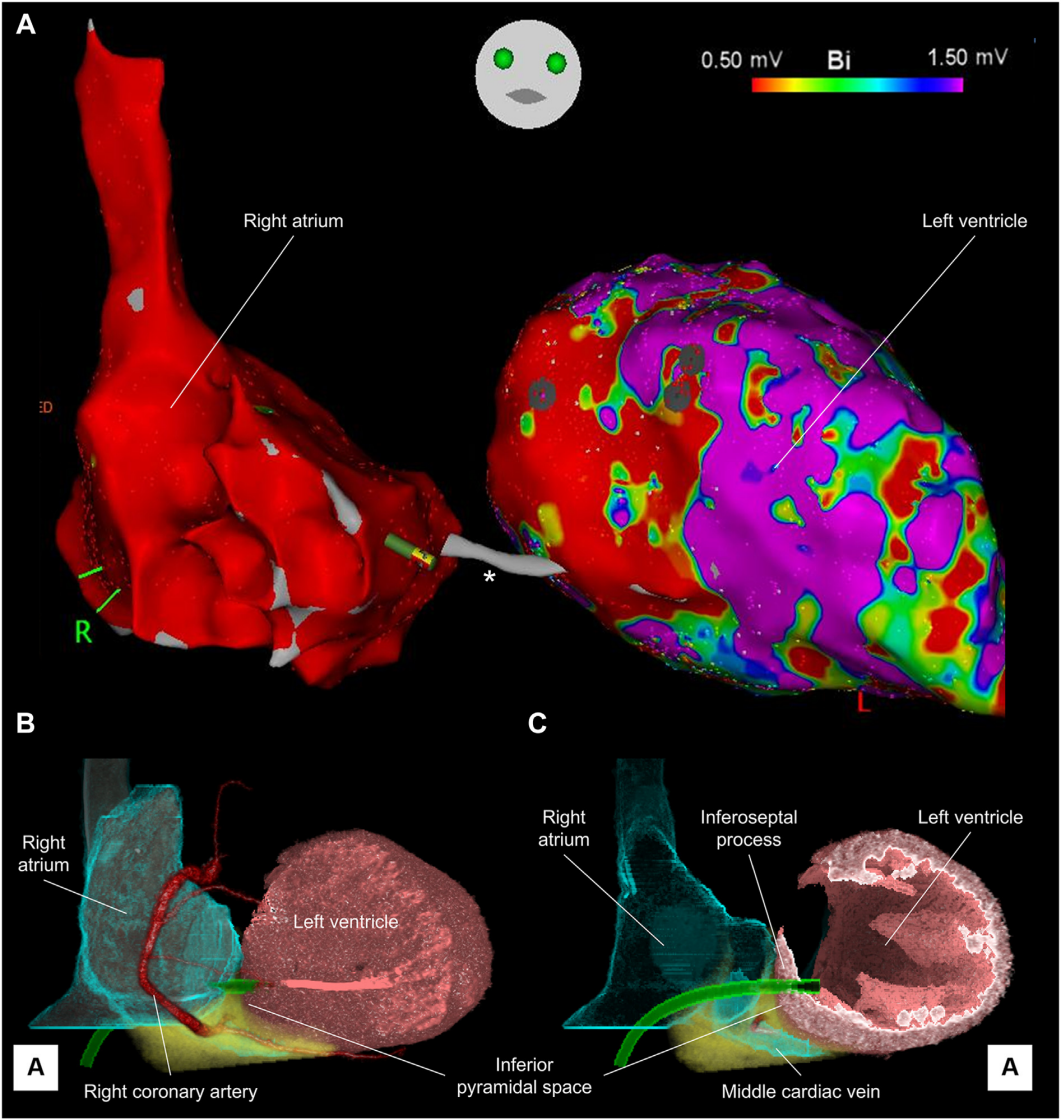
Voltage map of the left ventricle. A: After the right atrium to left ventricular puncture, bipolar voltage map of the left ventricle was created, showing dense scar at basal septum. B and C: show corresponding volume-rendered images viewed from the frontal direction, reconstructed from the cardiac computed tomography. A virtual sheath is reconstructed in *green*. The special gap between the right atrium and left ventricle in the voltage map (*asterisk*) consists of the right atrial wall, inferior pyramidal space, and inferoseptal process of the left ventricle.
